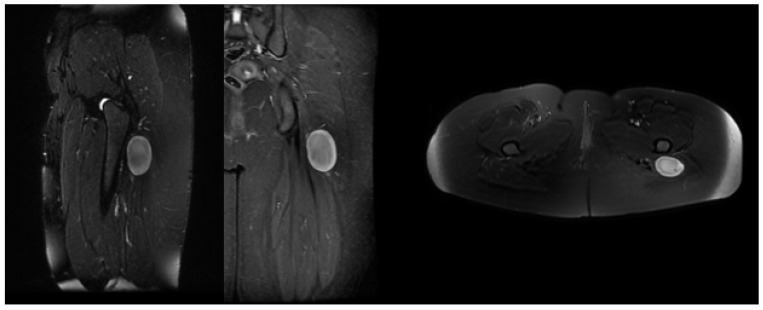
Sagittal, coronal, and axial T2-weighted MRI images of a 29-year-old female patient demonstrating imaging findings consistent with schwannoma. The patient presented with preoperative paresthesia and pain, which regressed following complete surgical excision of the lesion in the postoperative period.
